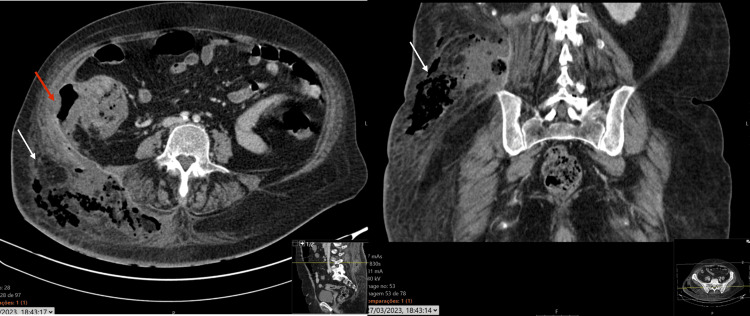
CT scan of the abdomen and pelvis The white arrows show the area of necrotizing fasciitis, with gas bubbles in the subcutaneous cellular tissue.
